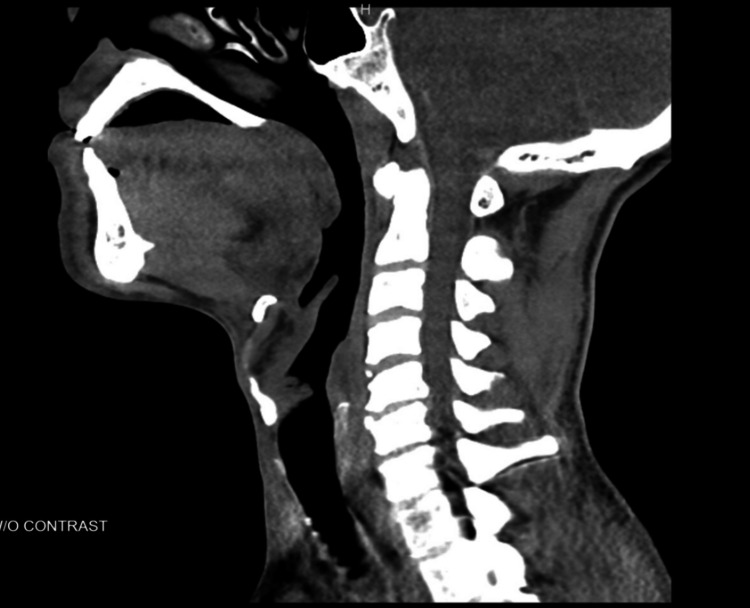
CT of cervical spine without contrast showing no acute fracture or subluxation. Cervical lordosis was maintained, and the posterior margins of the vertebral bodies were aligned. The vertebral bodies heights were maintained, with no evidence of atlantooccipital dislocation. No prevertebral soft tissue edema was noted. There were biapical blebs and multilevel degenerative changes of the cervical spine, characterized by varying degrees of disc space narrowing, marginal osteophytosis, endplate sclerotic or cystic changes, and facet hypertrophy. CT: Computed tomography
